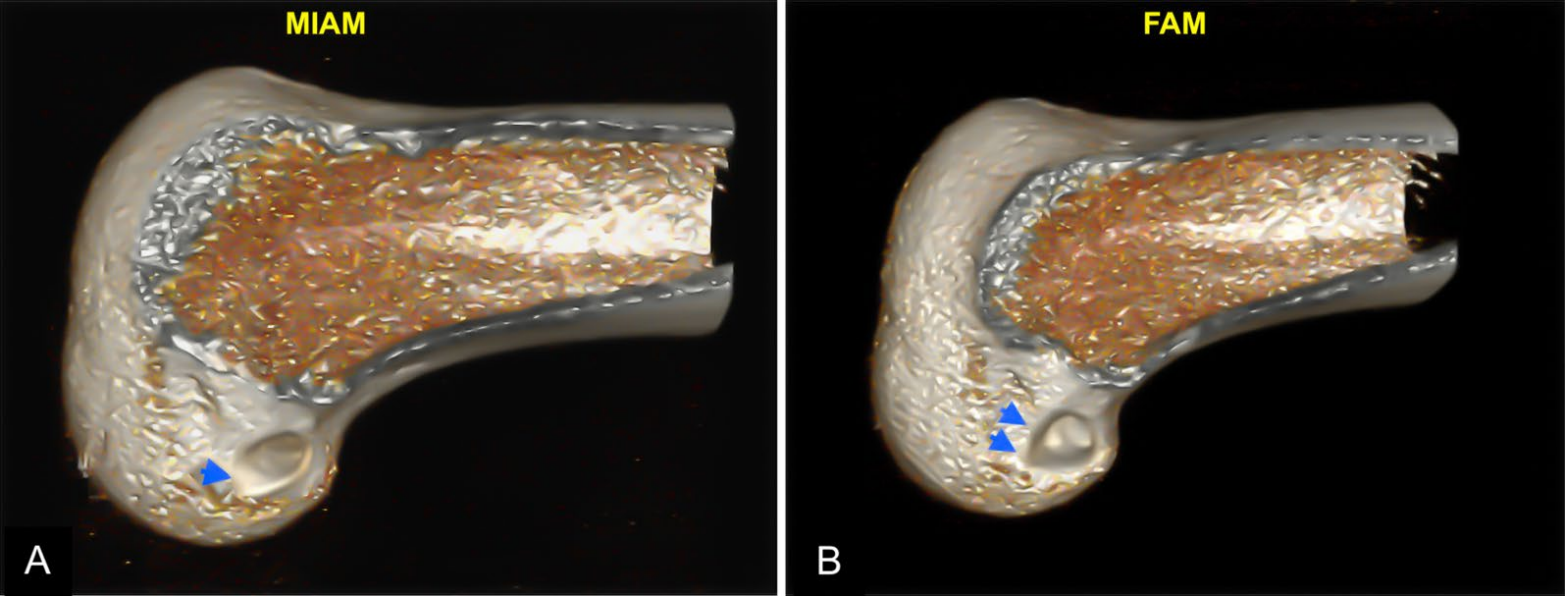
Three-dimensional CT images of entry apertures in the femoral tunnels. A The oval entry aperture of the femoral tunnel created through the MIAM portal (single arrowhead), and B a near-round entry aperture of the femoral tunnel created via the FAM portal (double arrowheads). CT, computed tomography; FAM, far anteromedial portal; MIAM, modified inferior anteromedial portal
